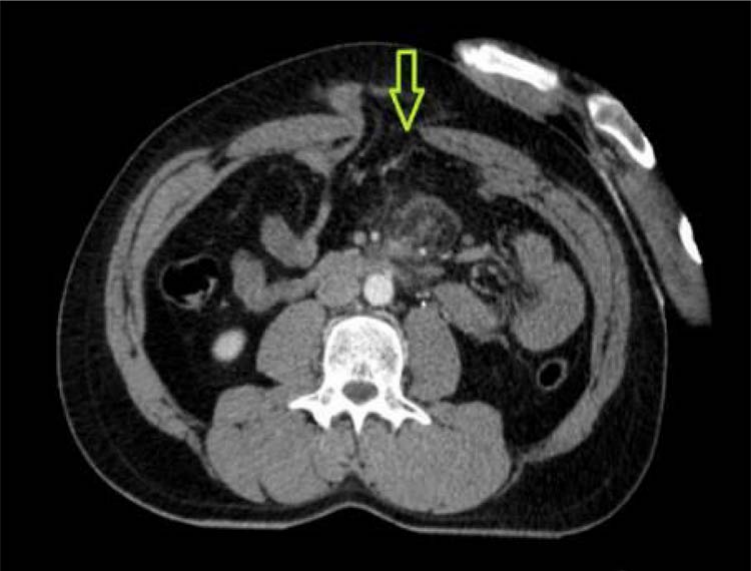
CT abdomen showing abdominal wall defect (green arrow).
